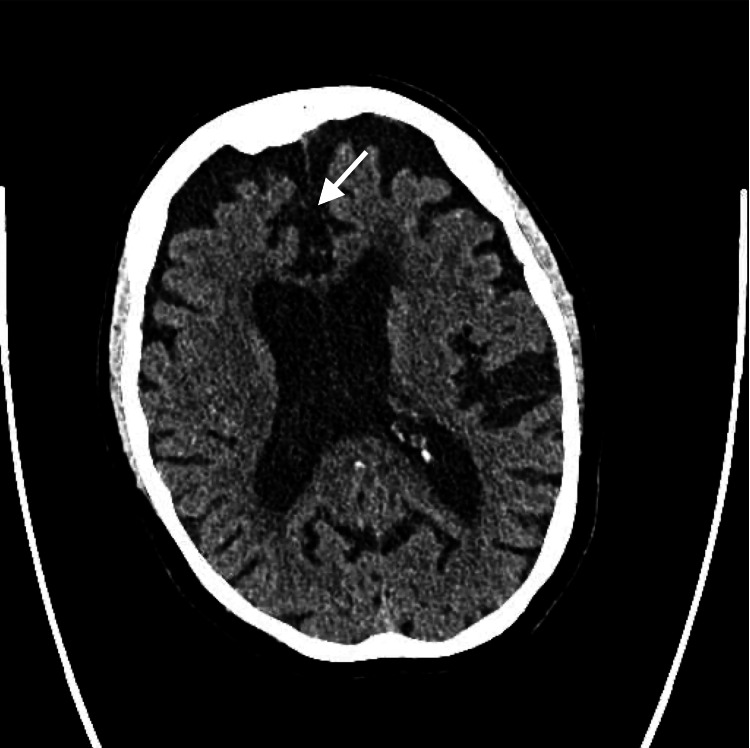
CT scan of the head The image shows cortical atrophy (white arrow).
